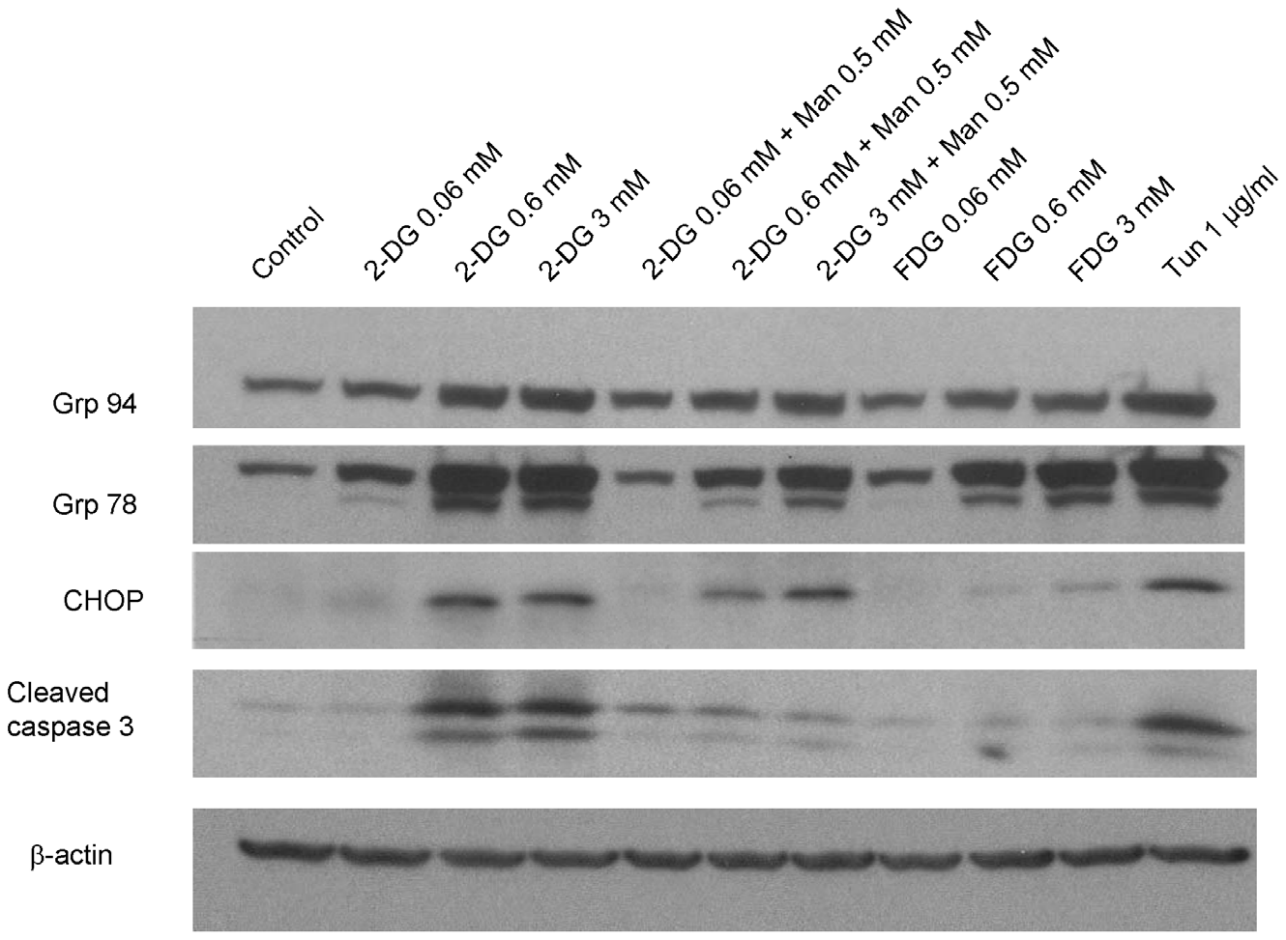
2-DG induces HUVEC unfolded protein response (UPR) and UPR mediated apoptosis. HUVECs were treated with 2-DG, with or without mannose, and 2-FDG for 24 hours, and immunoblotting was performed of cell lysates. 2-DG induced upregulation of Grp 94 (first panel) and Grp 78 (second panel) chaperone proteins and markers of the unfolded protein response. These effects were reversed by mannose (0.5 mM). CHOP/GADD 153, a transcription factor involved in ER stress mediated apoptosis, was potently upregulated by 2-DG (0.06, 0.6, and 3 mM) and partially reversed by mannose (third panel). CHOP/GADD 153 induction was associated with increased levels of cleaved caspase 3 in HUVECs treated by 2-DG (fourth panel). 2-FDG (0.06, 0.6, and 3 mM) induced a mild to moderate UPR response, especially at higher concentrations. However, CHOP/GADD 153 was not significantly induced, and levels of cleaved caspase 3 were not increased. Tunicamycin was used as positive control of the induction of UPR.
